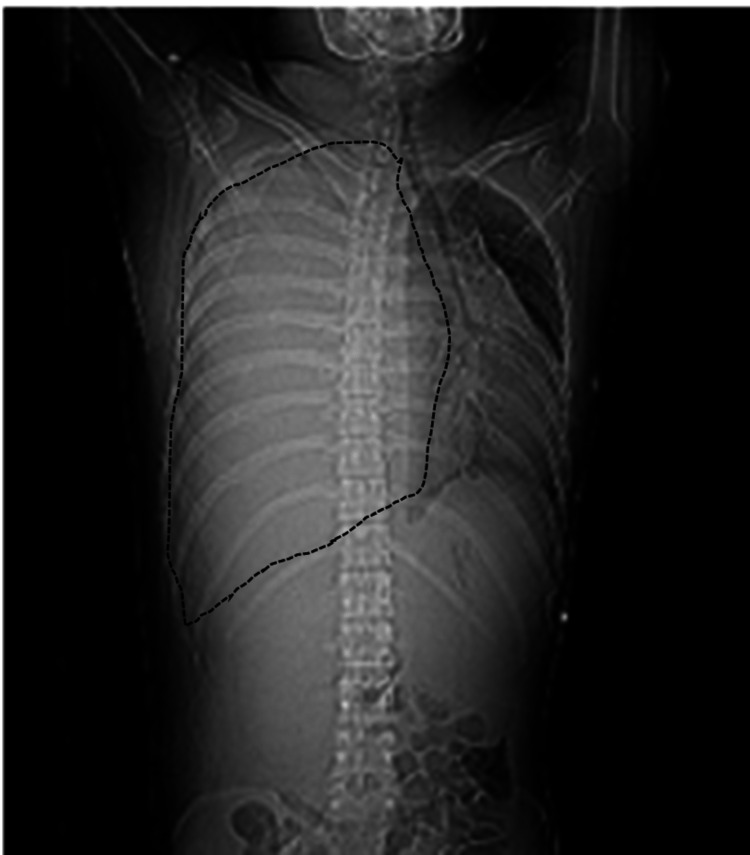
Chest x-ray (CXR) CXR Shows complete opacification of the right hemithorax with a marked leftward shifting of the trachea and the heart (black dotted line)
